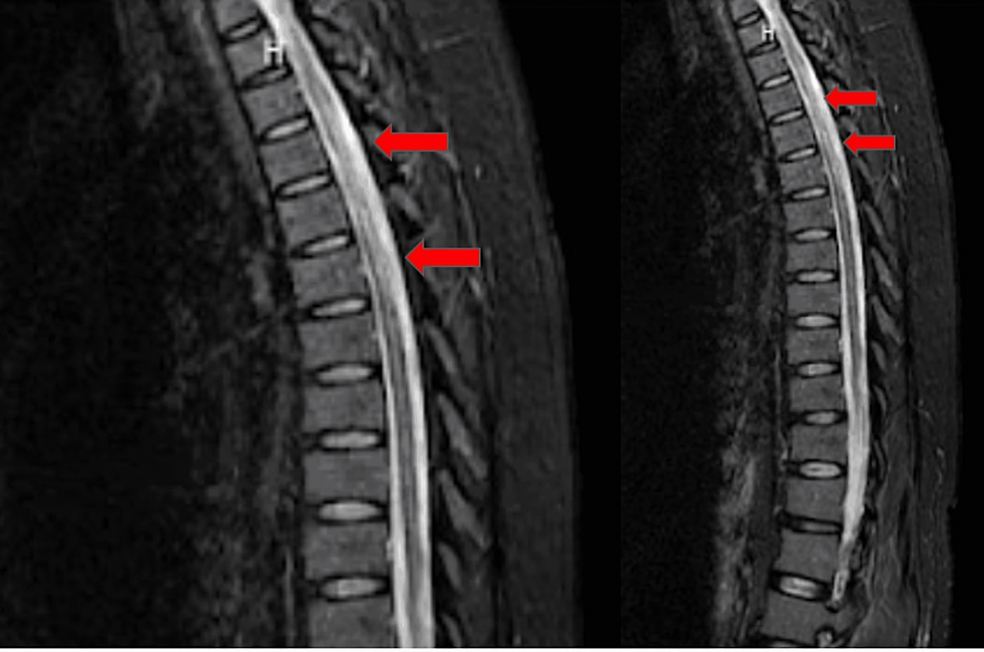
Magnetic resonance imaging of the thoracic spine showing signal abnormalities of the cord involving the central aspect with inflammatory etiologies consistent with neuromyelitis optica (red arrows).
